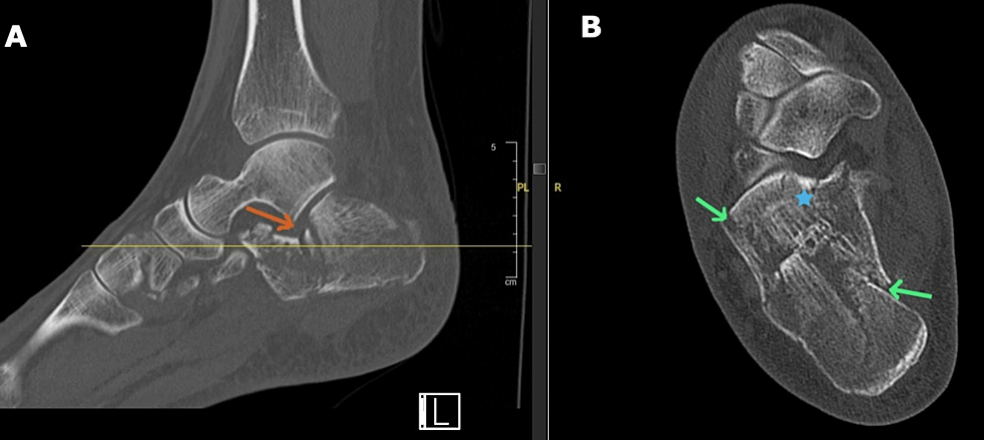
Image A is a sagittal CT image reconstruction with the orange arrow pointing to the intra-articular fracture with depression of the joint surface. The thin yellow line represents the level of the cut for the corresponding axial image on the right. Image B is an axial CT scan showing a displaced intra-articular calcaneal fracture. The green arrows point to the primary fracture line while the blue star represents the superomedial or constant fragment
